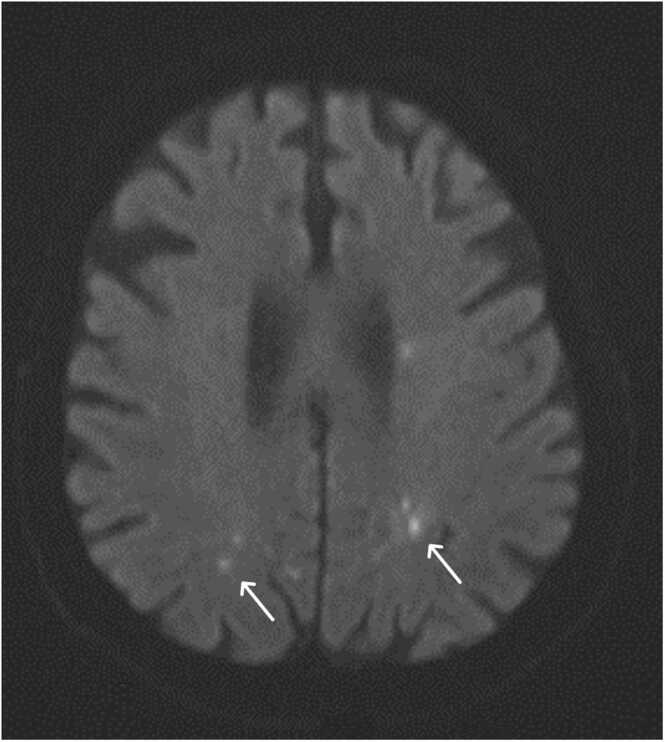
Horizontal MRI sections demonstrating selections of multiple brain lesions.
